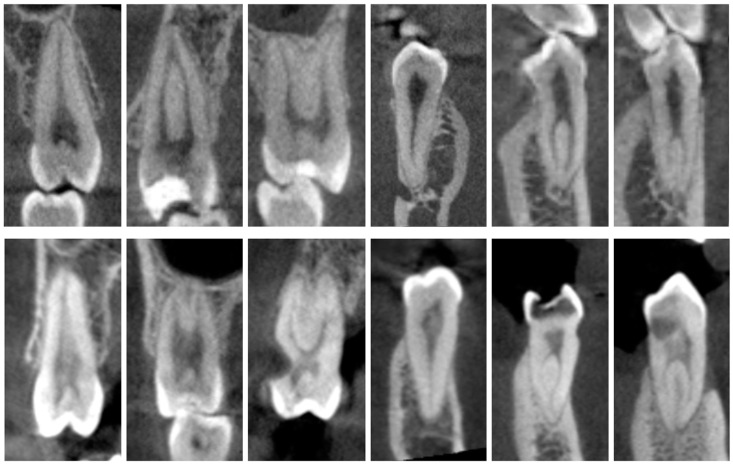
Representative CBCT images showcasing the root canal configurations in both maxillary and mandibular molars from the Jamaican (top) and Portuguese (bottom) subpopulations.
